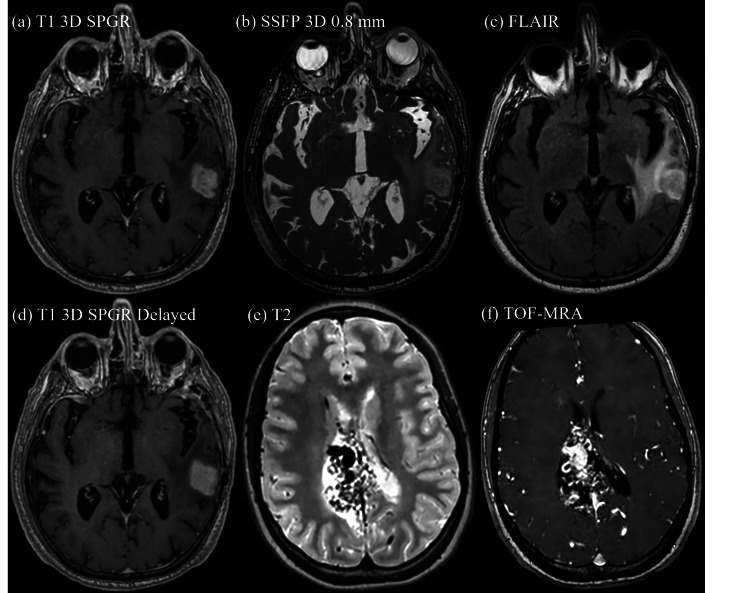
Representative images for JSUMC Synaptive MRI protocols: (a–d) Posterior lateral temporal lobe glioblastoma. (e–f) Right occipital lobe arteriovenous malformation. Image credit: Michael Chaga JSUMC: Jersey Shore University Medical Center
